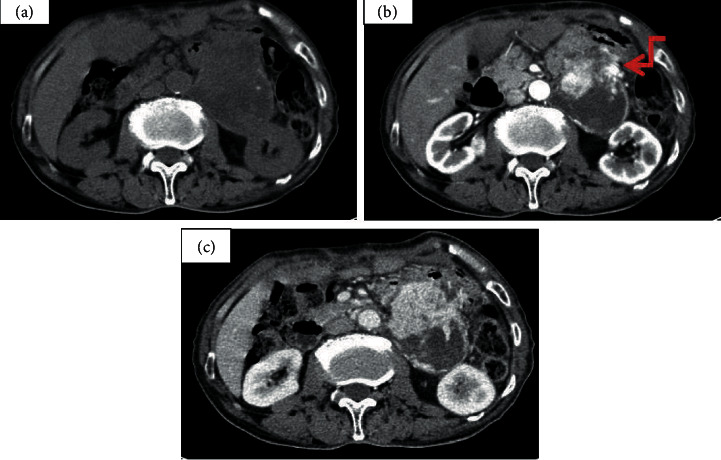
One case of a surgery confirmed retroperitoneal paraganglioma in a 77-year-old male that located in the left paraaortic area. (a) Nonenhanced CT scan presented a low heterogeneity density with an irregular shape tumour size of about 97 mm × 69 mm. The presence of multiple-size varied cystic degeneration and speckled calcification. (b, c) Dynamic enhanced CT scans revealed an obvious heterogeneously enhanced tumour for solid components. Peritumor and intratumor blood vessels (arrow). CT values were 32, 154, and 134 Hounsfield units (HU), respectively, in the nonenhanced, arterial, and venous phases.
